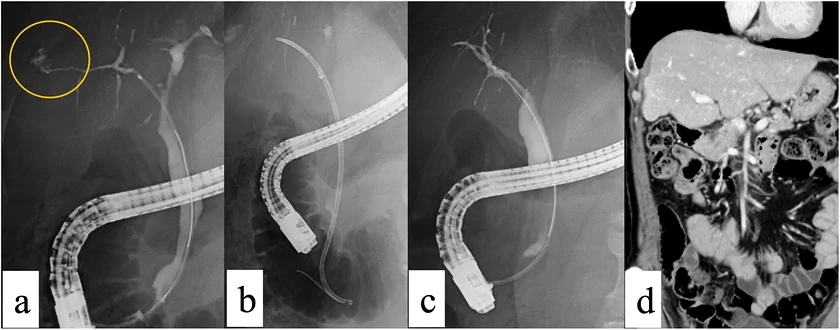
Additional internalization and treatment completion. (a) Fluoroscopic image at endoscopic retrograde cholangiopancreatography (ERCP) approximately 3 months after transpapillary/transfistular (TP/TF): the circle indicates low‐grade leakage from B7, representing improvement from the initial high‐grade leak. (b) A 7‐Fr plastic stent was placed in the B7 bile duct to complete internalization. (c) ERCP approximately 8 months after TP/TF: cholangiography revealed no biliary leakage, and the stent was removed. (d) Final computed tomography (CT) after stent removal: complete resolution of both bilomas was confirmed.
